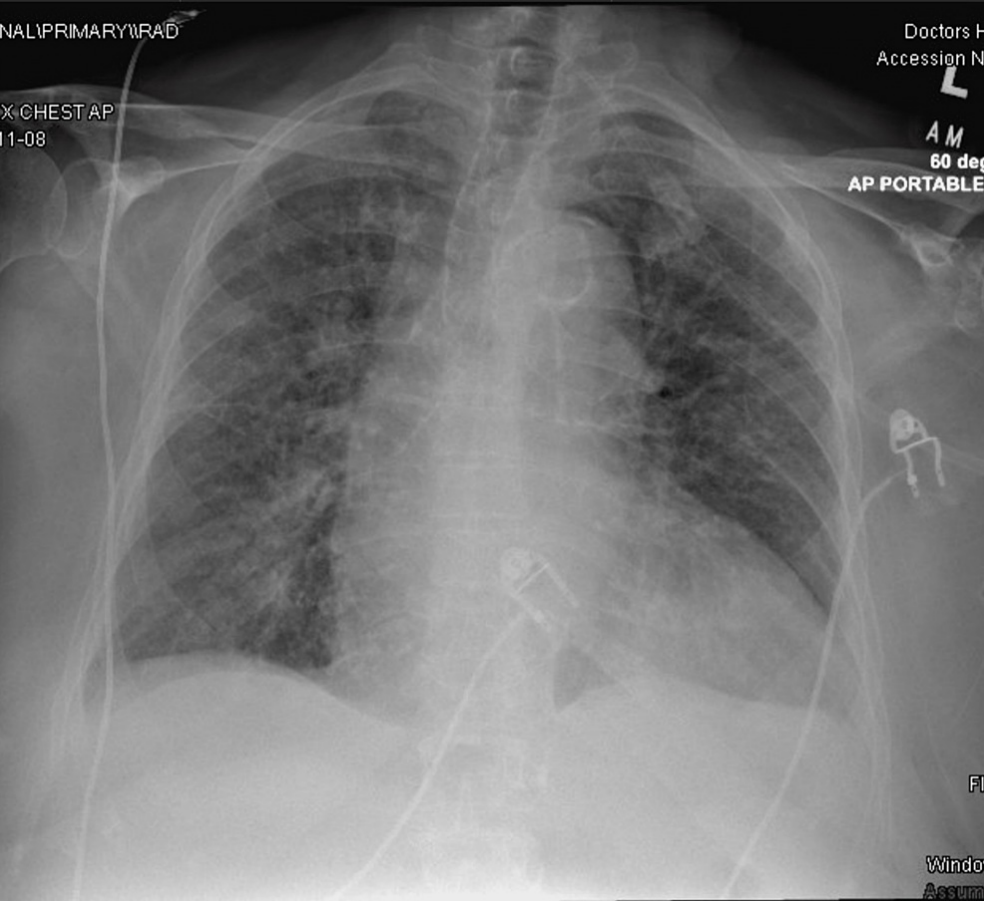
Chest X-ray showing increased interstitial markings suggestive of either atypical pneumonia versus pulmonary edema versus interstitial pneumonitis
